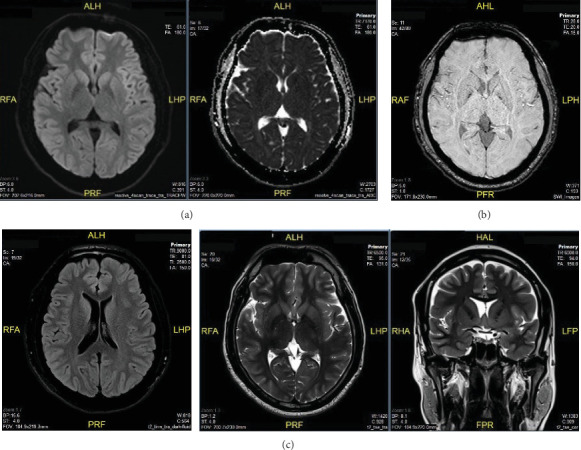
Brain MRI findings. (a) DWI-ADC sequences show no ischemic stroke. (b) SWI sequence shows no intracranial hemorrhage. (c) FLAIR/T2W sequences show no characteristic findings of hyperammonemic encephalopathy.
